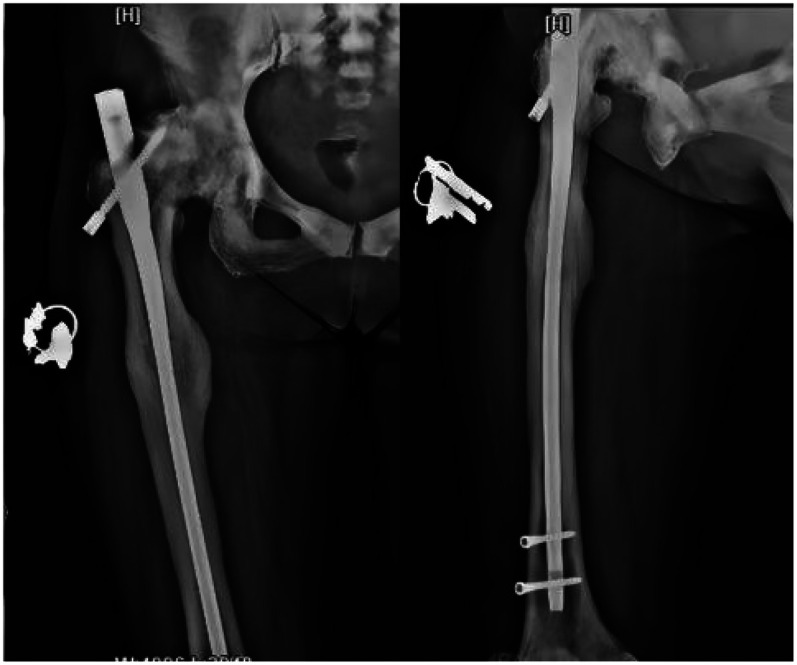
Follow-up x-rays taken one year after surgery showed good fracture healing and no abnormalities in the internal fixation device.
